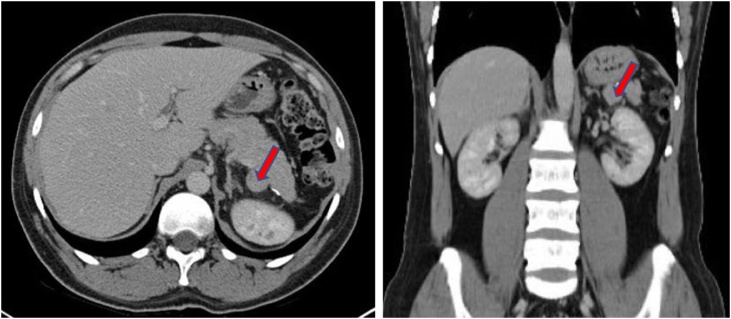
Enhanced CT scan of abdomen showing a well-defined rounded hyperattenuating soft tissue lesion inseparable from the posterior wall of the pancreatic tail measures 2 cm in diameter.
